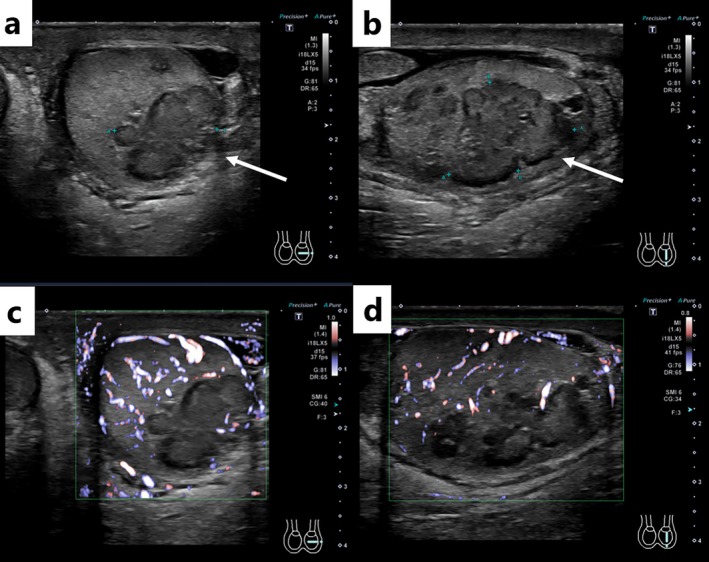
Ultrasonographic findings of the left testis. Ultrasonography reveals a mass in the left testis appearing as multiple fused nodules (a, b; arrow). The borders are well‐defined, the margins are hypoechoic, and no detectable blood flow is noted (c, d).
